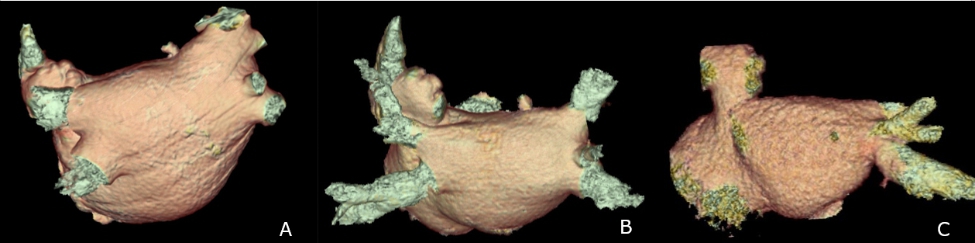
Cardiac CT with 3D reconstruction showing progressive reduction of left atrial volume. (A) LA volume 141 ml before ablation. (B) LA volume 90 ml after first ablation procedure. (C) LA volume 51 ml after third ablation procedure with occlusion of the left PVs
